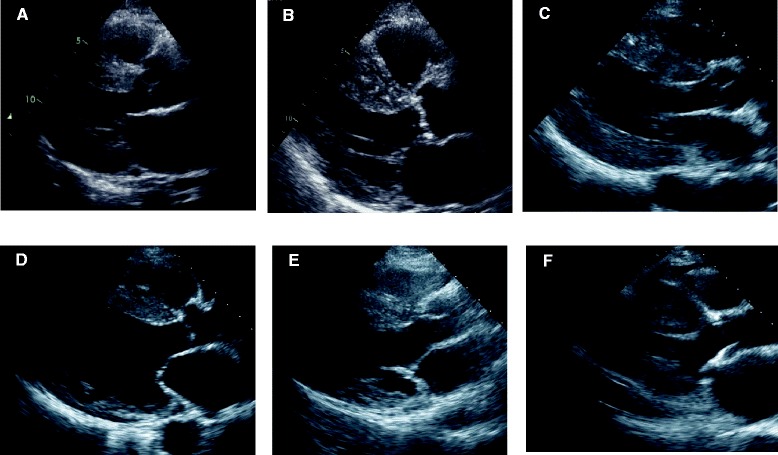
Long-axis two-dimensional echocardiograms (diastole phase) of patients with cardiomyopathies. a: a cardiac amyloidosis patient with concentric left ventricular (LV) hypertrophy, b: a cardiac amyloidosis patient with asymmetric septal hypertrophy (ASH) at first glance (but actually concentric hypertrophy rather than ASH if moderator band is removed), c: a cardiac Fabry patient with concentric LV hypertrophy, d: a cardiac Fabry patient with ASH (in a terminal stage patient with Fabry disease, LV systolic dysfunction with localized thinning of the base of the LV posterior wall is seen.), e: a hypertrophic cardiomyopathy (HCM) patient with ASH, f: a HCM patient with concentric LV hypertrophy at first glance
